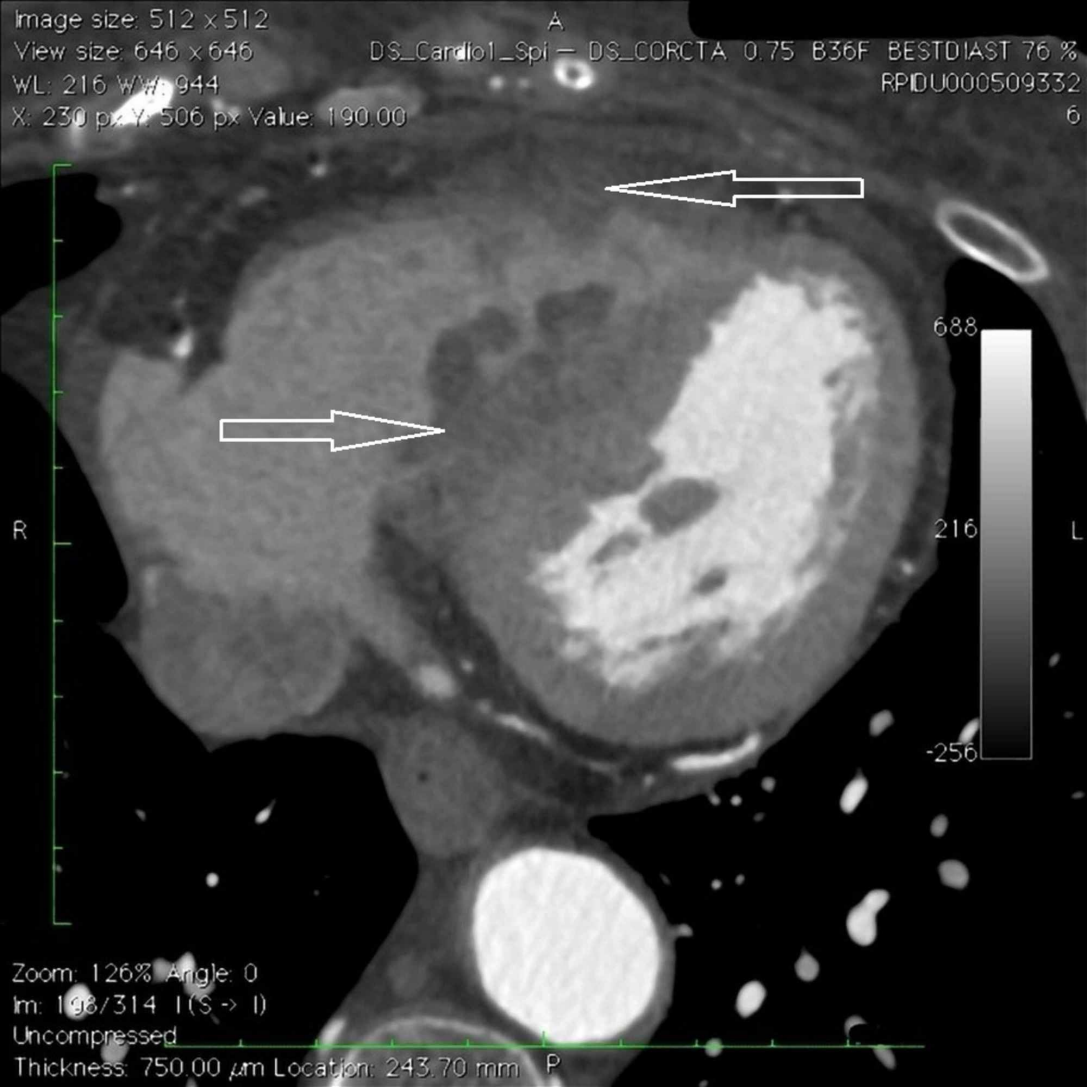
Computed tomography (CT) scan of the heart Axial plane contrast-enhanced.
